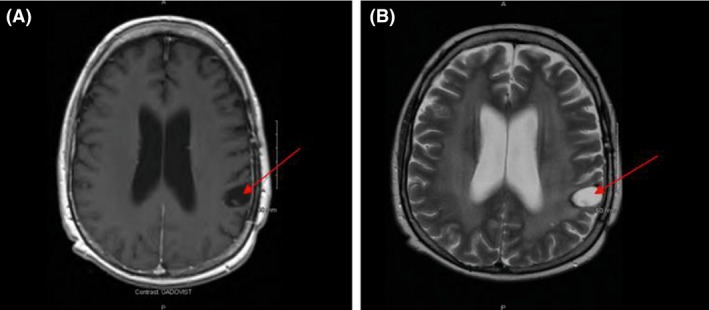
MRI brain showing residual small CSF filled cystic space at the site of previous neoplastic mass (Red arrows).
